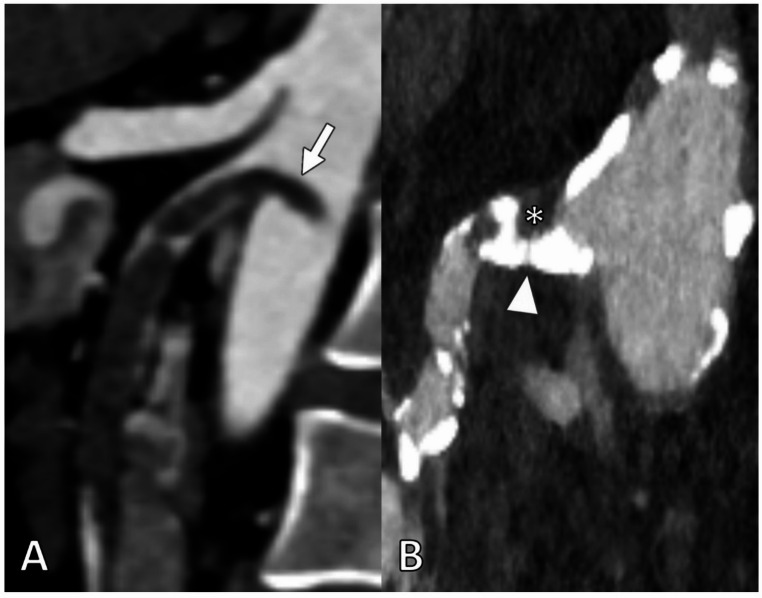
CT angiography in parasagittal views revealed two distinct pathological entities of AAOMI. (A) shows an embolic occlusion of the superior mesenteric artery, with the embolus protruding into the aortic lumen (arrow). Note the absence of atherosclerotic lesions. In contrast, (B) demonstrates a highly calcified atherosclerotic stenosis (arrowhead) at the SMA ostium, complicated by superimposed thrombotic occlusion (asterisk) due to plaque rupture
